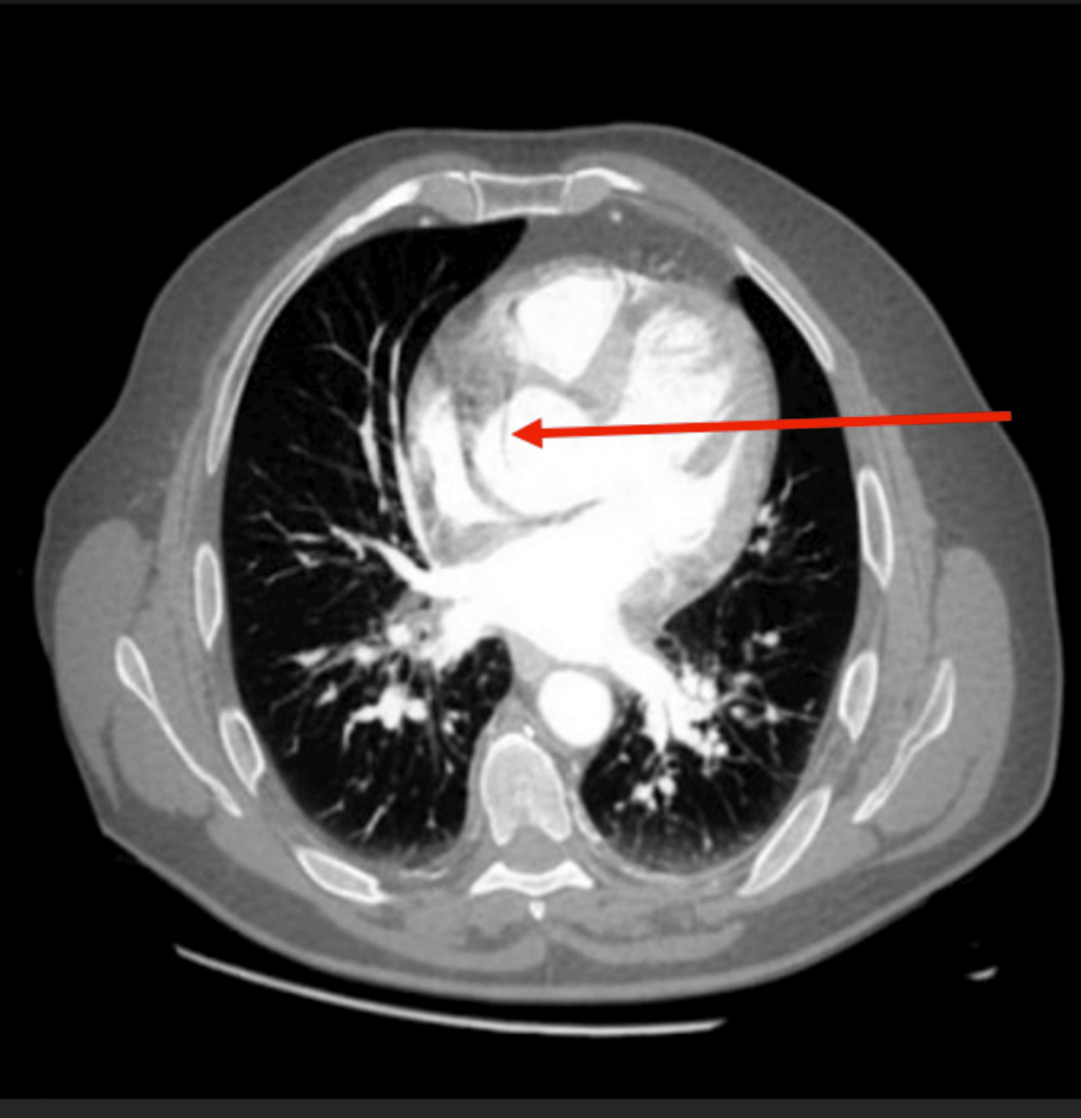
Axial contrast-enhanced CT aortography showing a dilated ascending aorta measuring 4.3 cm in diameter with an aortic dissection flap; the arrow indicates the site of dissection
